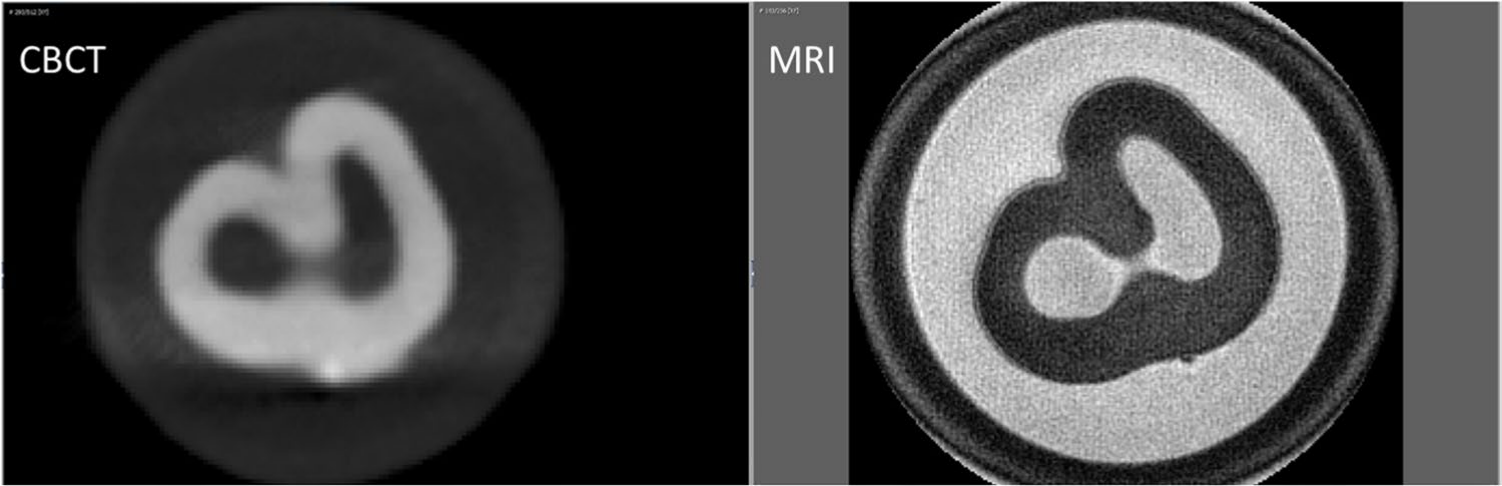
Tooth 48, tooth of 18-year-old male, transversal view. Left, CBCT imaging. Right, MRI imaging. The comparison clearly shows the better sharpness in the MRI images compared to the CBCT. In younger teeth, no changes were detectable within the dental pup on MRI either
